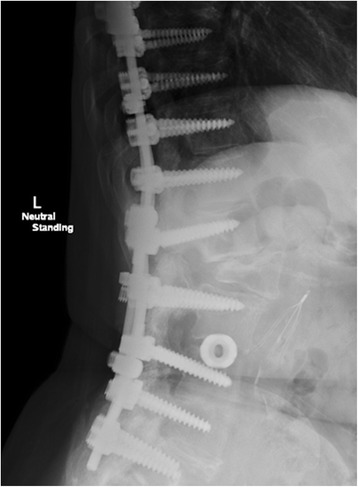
Lateral postoperative X-rays demonstrating rod repair
